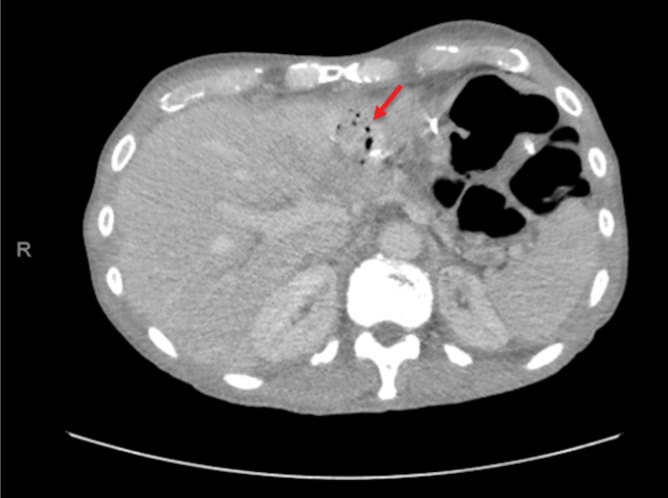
CT scan showing 7-cm collection with fluid and air (arrow) adjacent to the DJ and PJ. DJ, duodenojejunostomy; PJ, pancreatojejunostomy.
